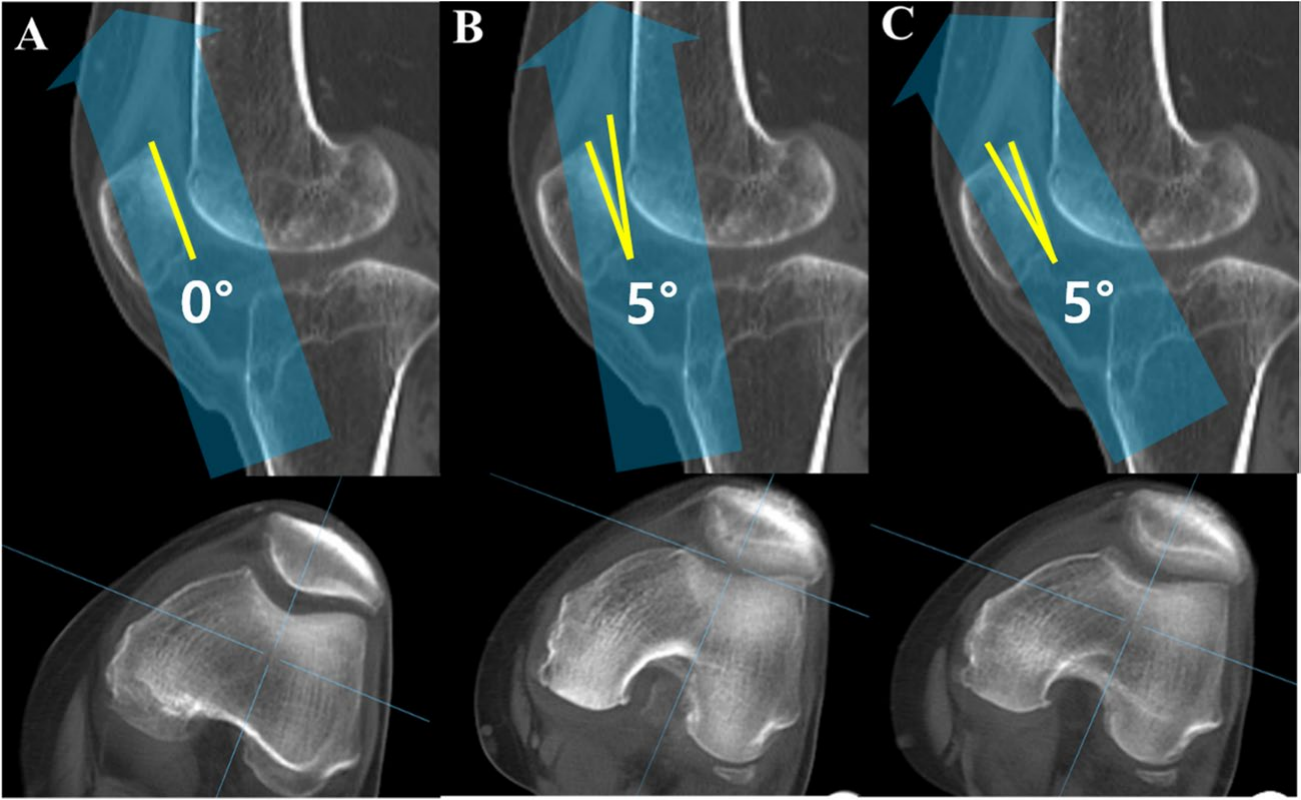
Examples of beam projection angle deviations and corresponding digitally reconstructed skyline images from CT scans. A Neutral tilt, B 5° downward tilt, and C 5° upward tilt. Blue arrows in each image indicate the direction of the beam projection, while yellow lines represent the patellar articular surface and the respective beam projection angle. As the beam projection angles deviate from the neutral tilt, there is a noticeable blurring of the patellar articular surface
